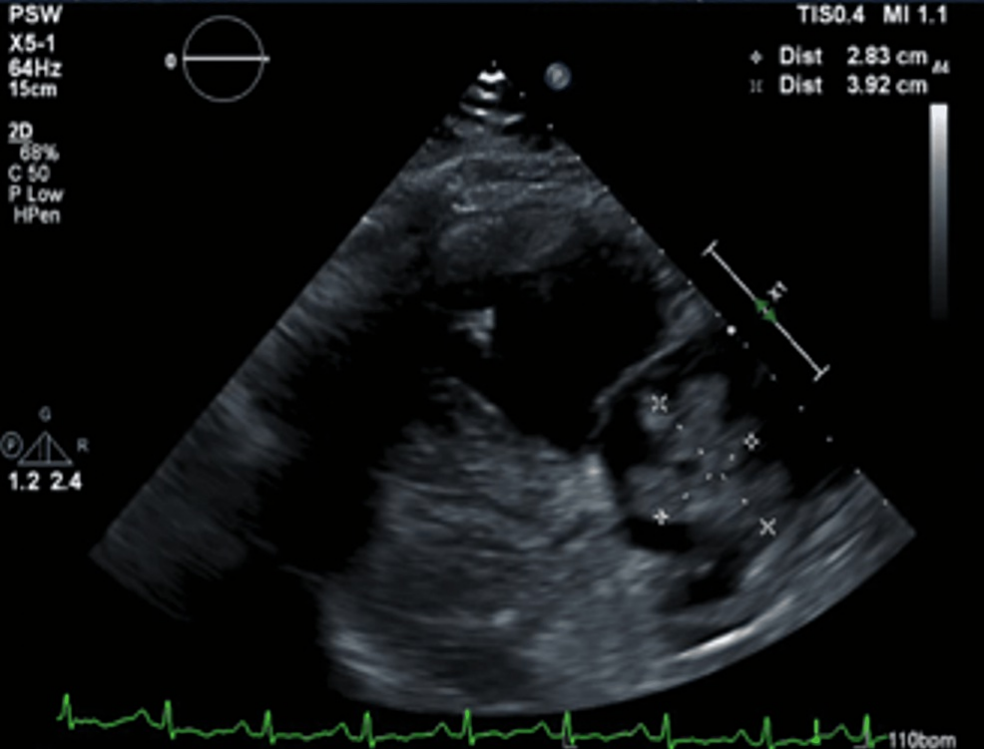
Transthoracic echocardiogram showing 2.83 cm × 3.92 cm right atrial thrombus
